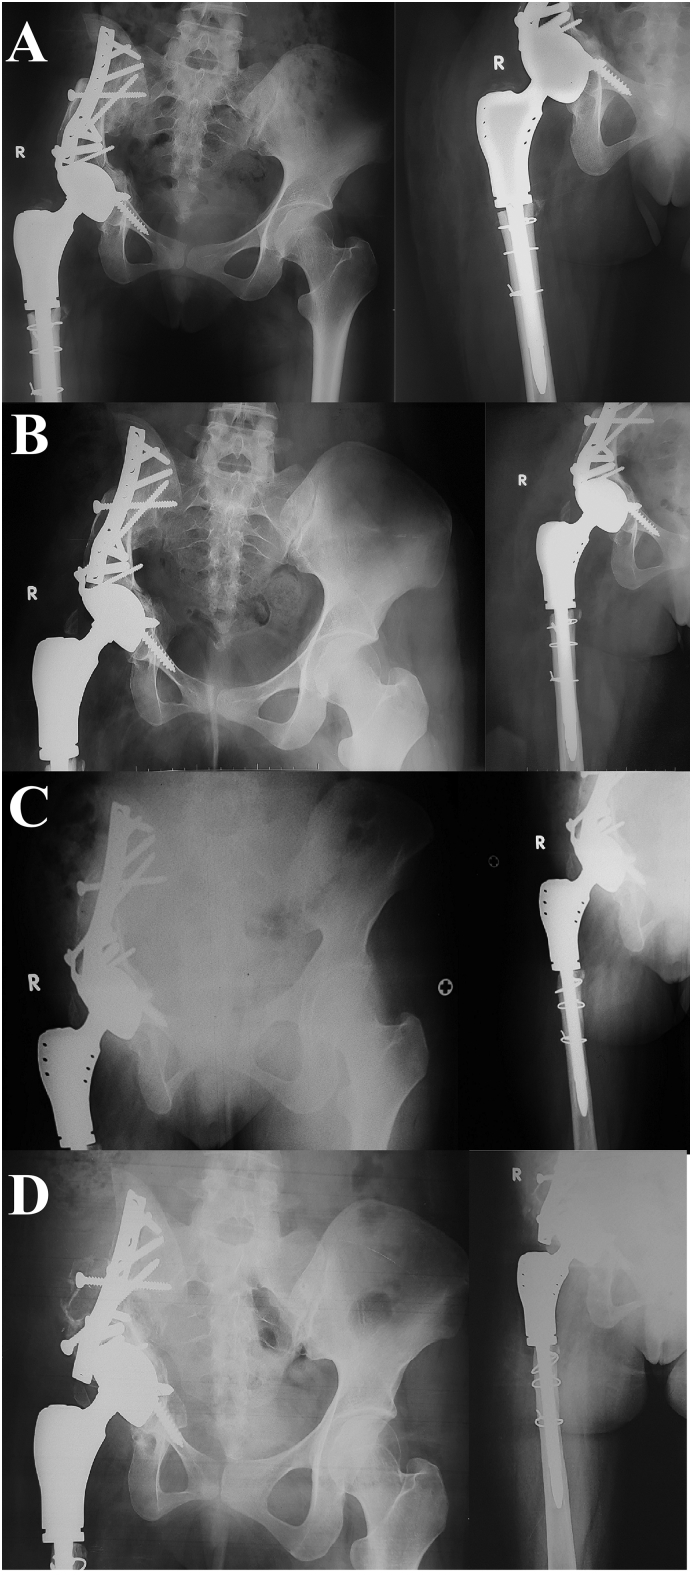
Postoperative and follow up radiographic evaluation (till the last follow up) showing proper positioning and stability of the implants. A: first postoperative follow up visit (January 2020). B: Follow up in June 2020. C: June 2021. D: last follow up in November 2022.
